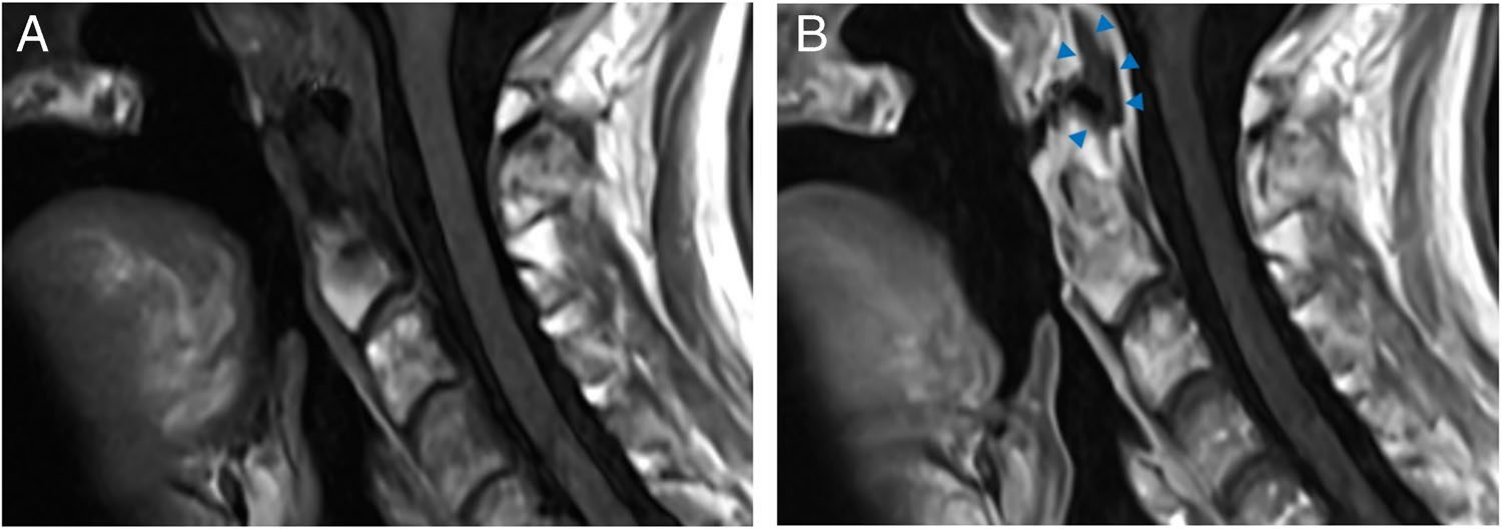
T1-weighted precontrast MR (A) and postcontrast MR (B) images demonstrate a peripherally enhancing fluid collection surrounding the odontoid process (arrowheads) with internal susceptibility corresponding to gas seen on CT. Cortical erosions, T1 hypointensity, and enhancement in the odontoid process are consistent with acute osteomyelitis
